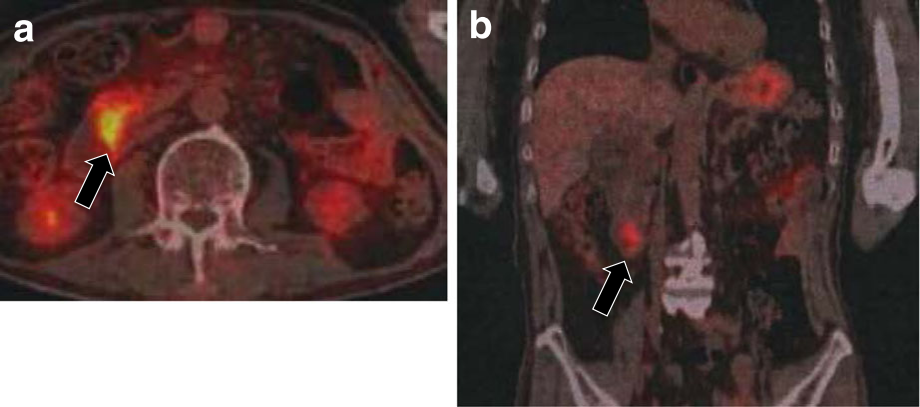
A 2-[^18^F]-fluoro-2-deoxy-d-glucose positron emission computed tomography revealed accumulation only at the site of primary duodenal tumor (a, b, *arrows*)
